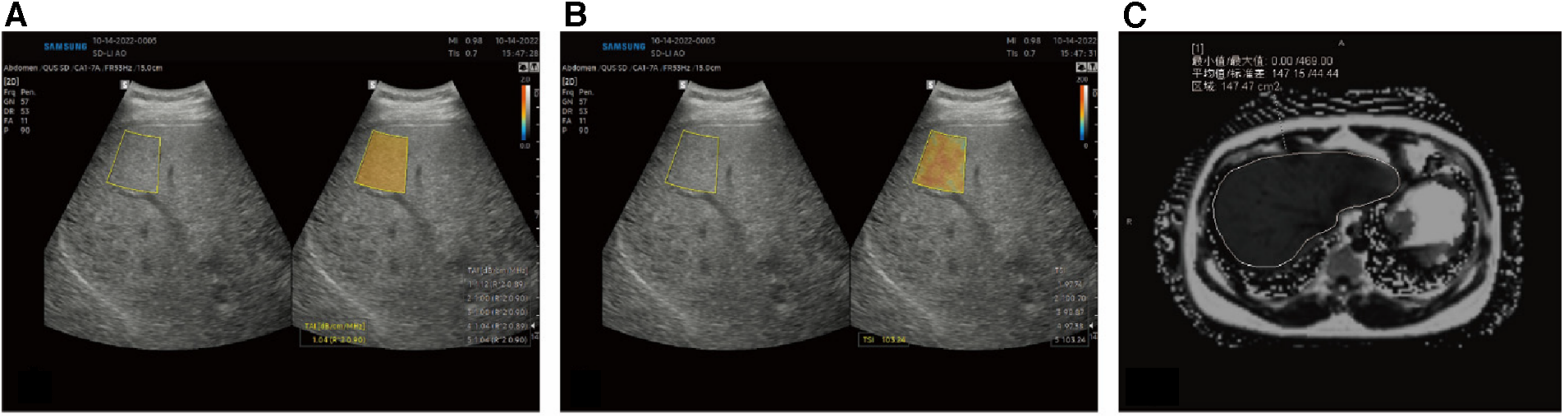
Quantitative analysis of liver fat in an obese Chinese male. Based on B-mode ultrasound image, the color-coded maps of TAI map (A) reflecting center frequency and TSI map (B) reflecting Nakagamia parameters are generated. (C) MRI-PDFF values were 14.72%.
